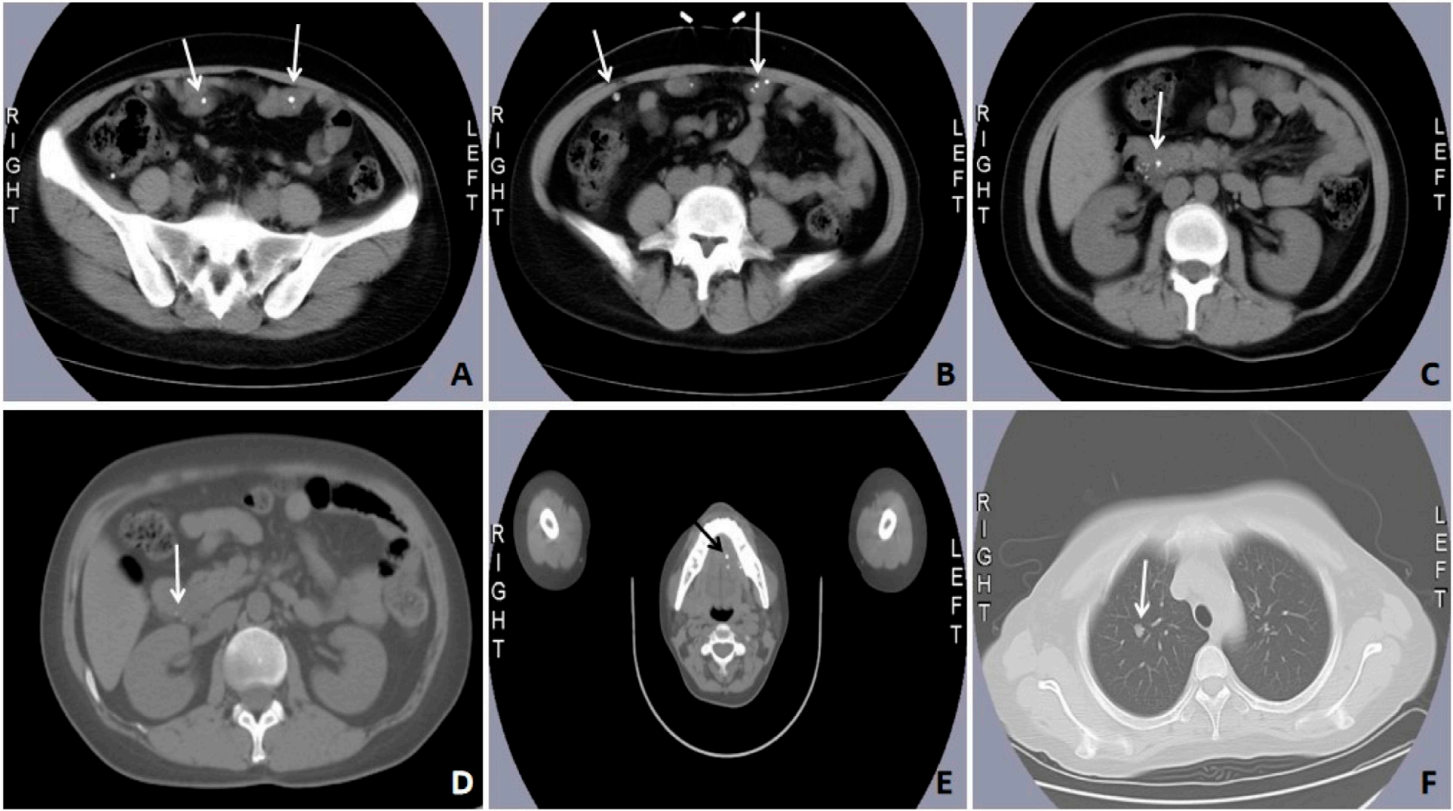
Computed tomography imaging and the positron emission tomography/computed tomography (PET/CT) scan of case #1. Abdominal CT showed multiple spotted calcifications in the intestine (A–C) and the head of the pancreas (D). Tomography/computed tomography (PET/CT) scan showed multiple spotted calcifications in the hard palate (E). Chest CT showed a soft tissue mass shadow in the lung (F) (marked by the white or black arrows).
